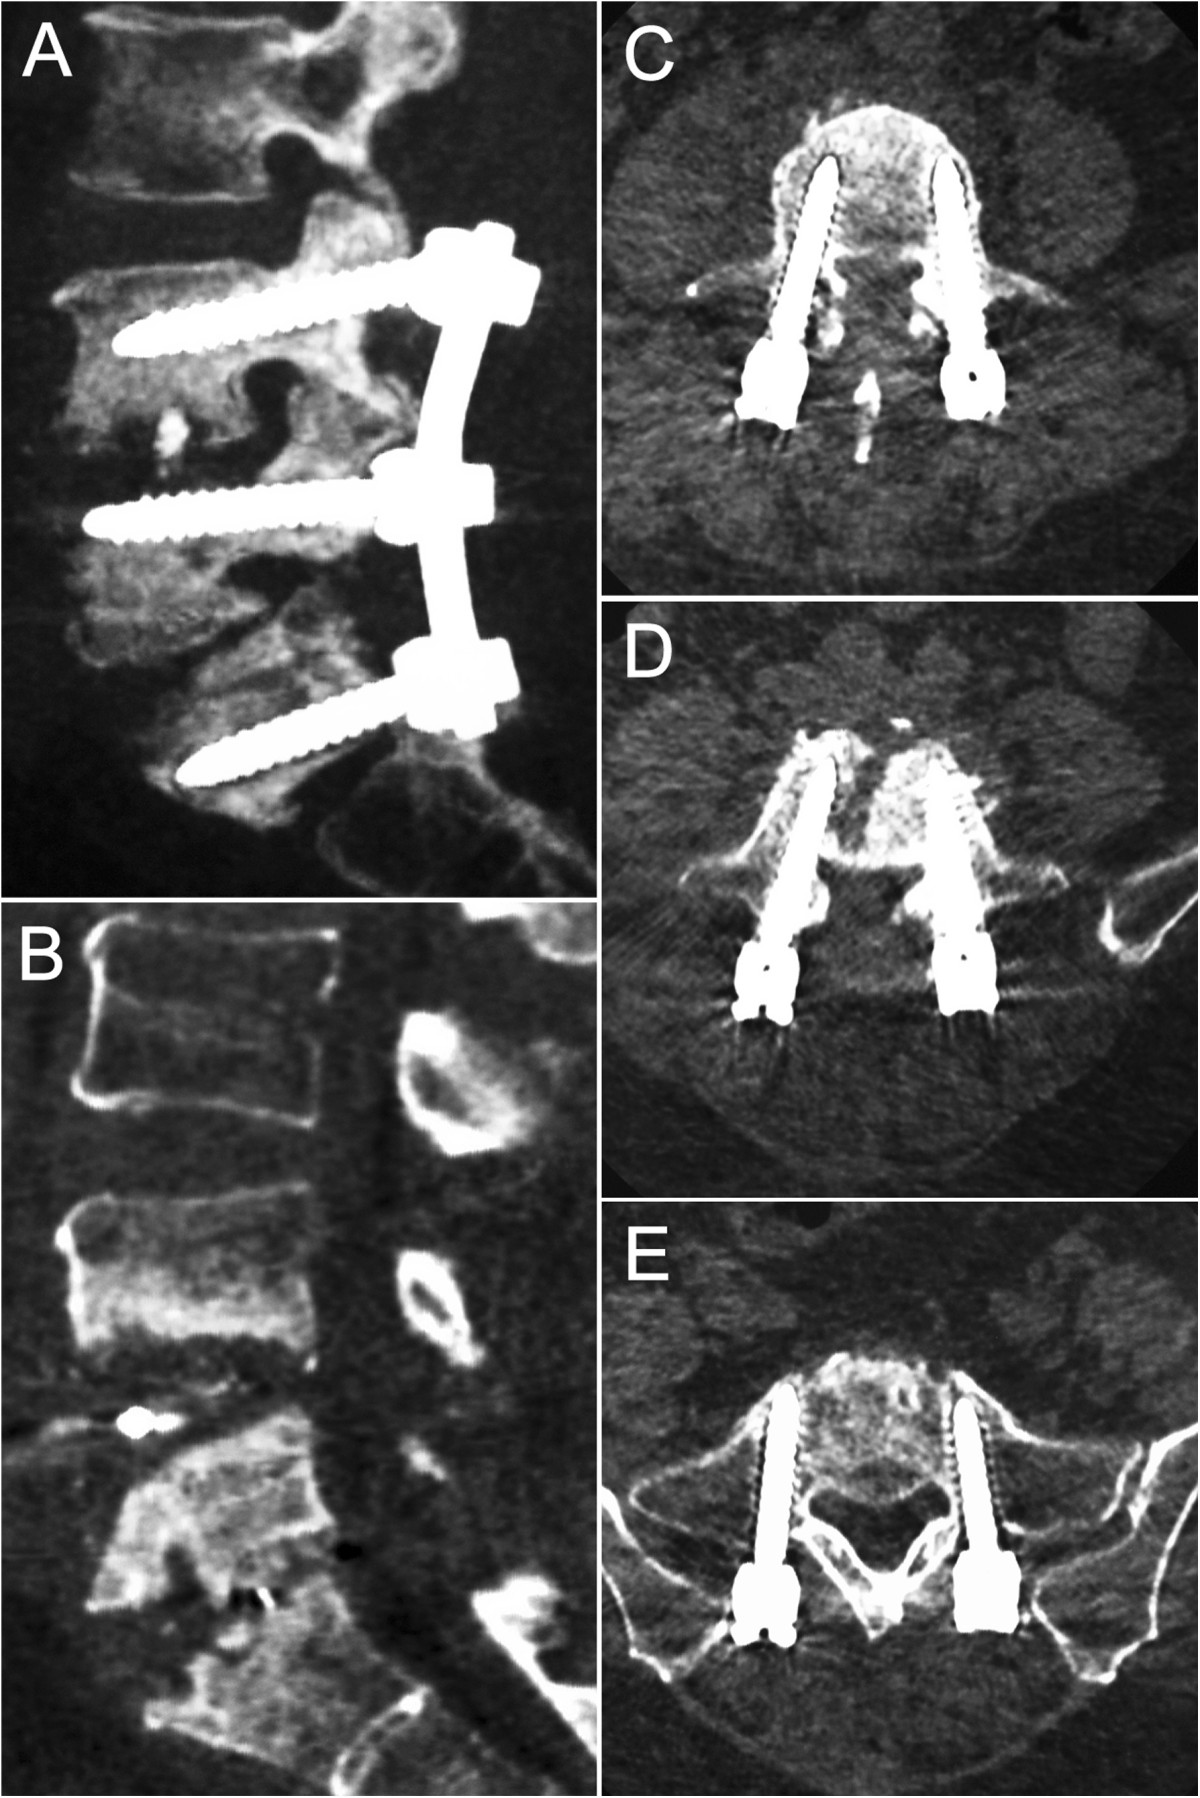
Osteolysis between L4 to S1. Sagital, Computed tomography (CT) revealing osteolysis between L4 to S1 (A, B). Note the gap around the pedicle screws in the vertebrae body(especially in C and E), and attenuation of the erector spinae is also evident in the axial view (C, D, E).
